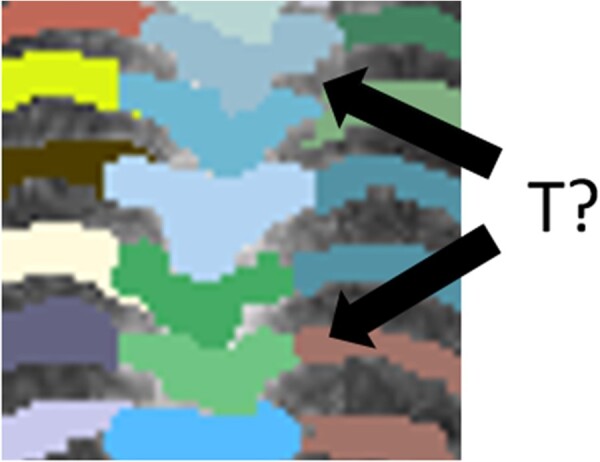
The challenges of patch-wise segmentation: mis-localization and classification of similar shaped structures. Organs with similar characteristics in close proximity are difficult to differentiate among each other. The figure shows an example of the thoracic portion in a posterior whole-body bone scintigraphy patch. ‘T?’ means the index of thoracic spine. It is difficult to distinguish the anatomy structure from this patch
